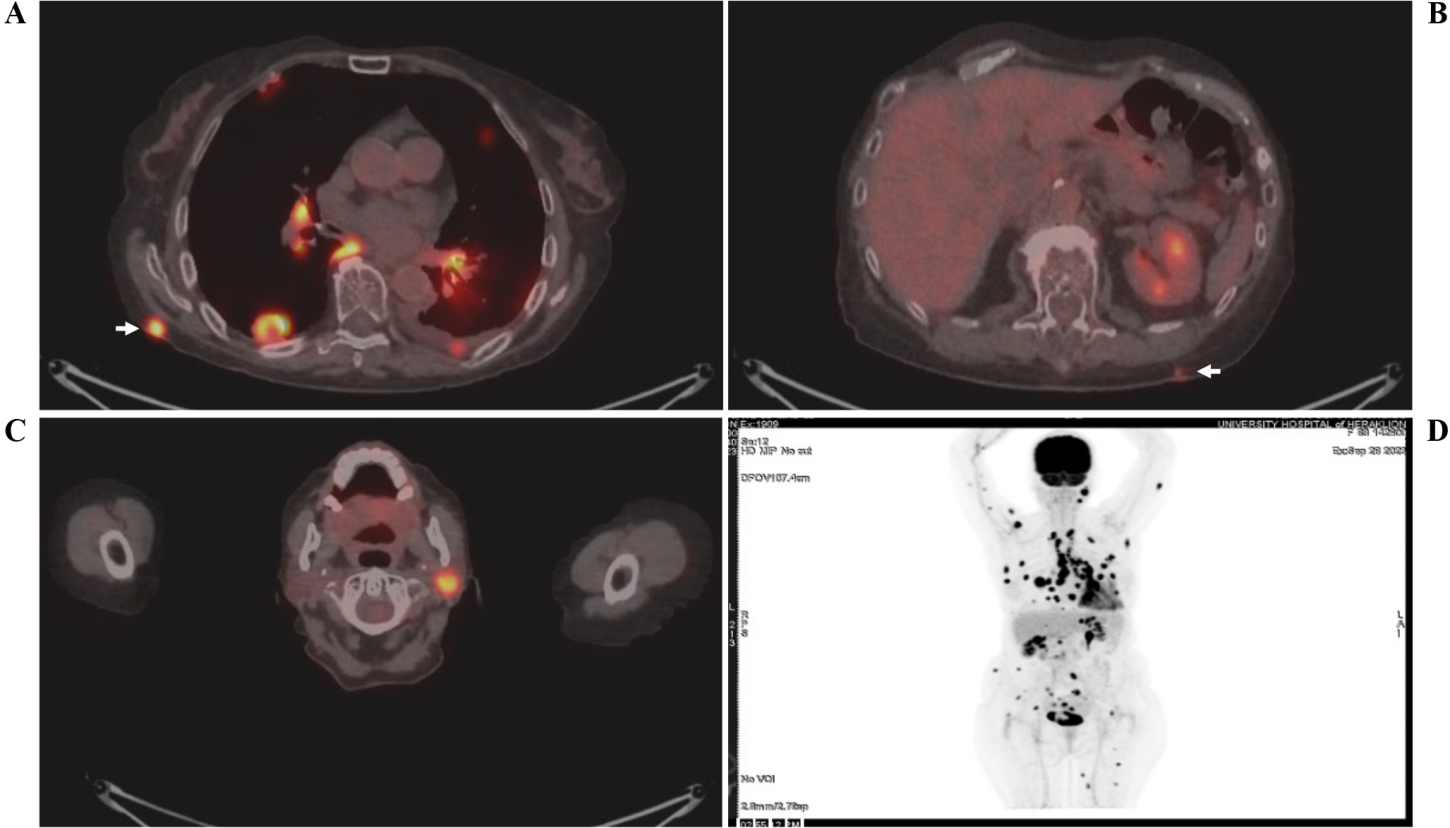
^18^F-FDG-PET/CT scan showing hypermetabolic uptake in the (A, B) skin (white arrows) and (C) left parotid gland. A whole-body scan (D) is also shown. The patient’s name and date of birth have been omitted for confidentiality reasons.
